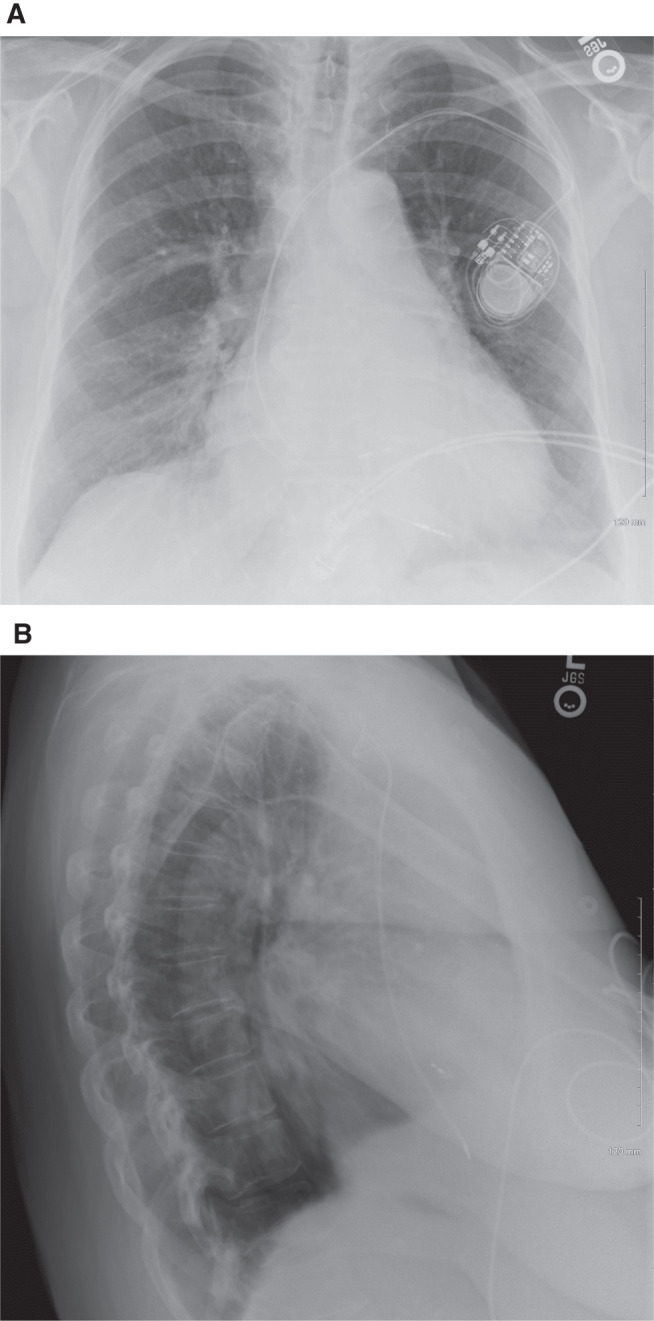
Postero-anterior (A) and lateral (B) postoperative chest X-rays showing satisfactory lead positioning.
